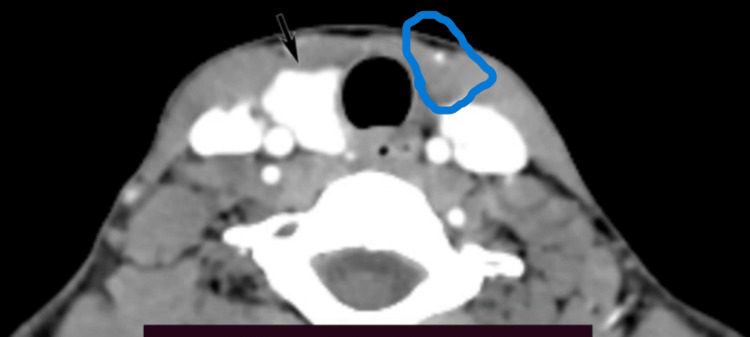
Axial Contrast-Enhanced CT Scan of the Neck Axial contrast-enhanced CT scan of the neck demonstrating the absence of the left thyroid lobe, with the blue line encircling the empty thyroid bed. The arrow indicates the right thyroid lobe, which is present CT: computed tomography
